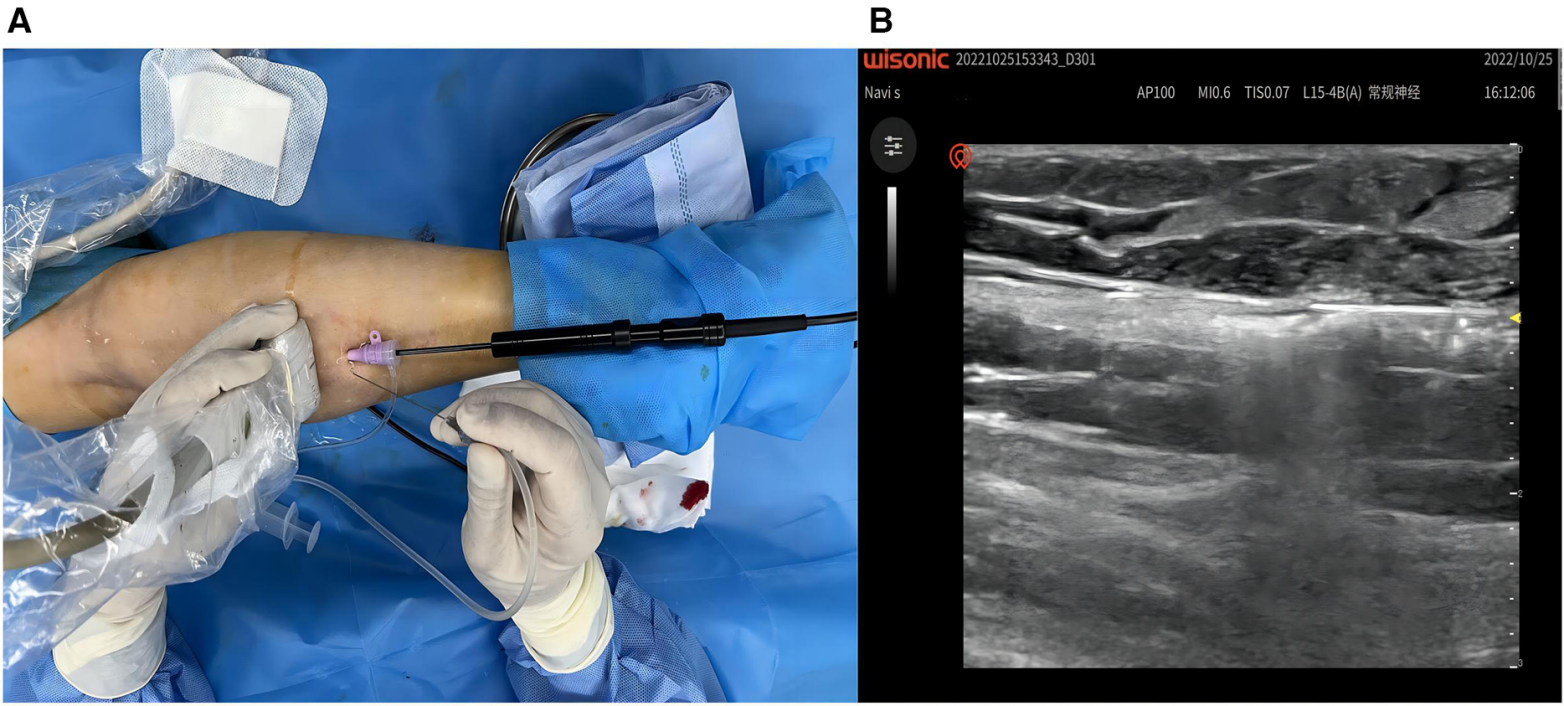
(A) Ultrasound-guided tumescent anesthesia. (B) Ablation under ultrasound monitoring.
